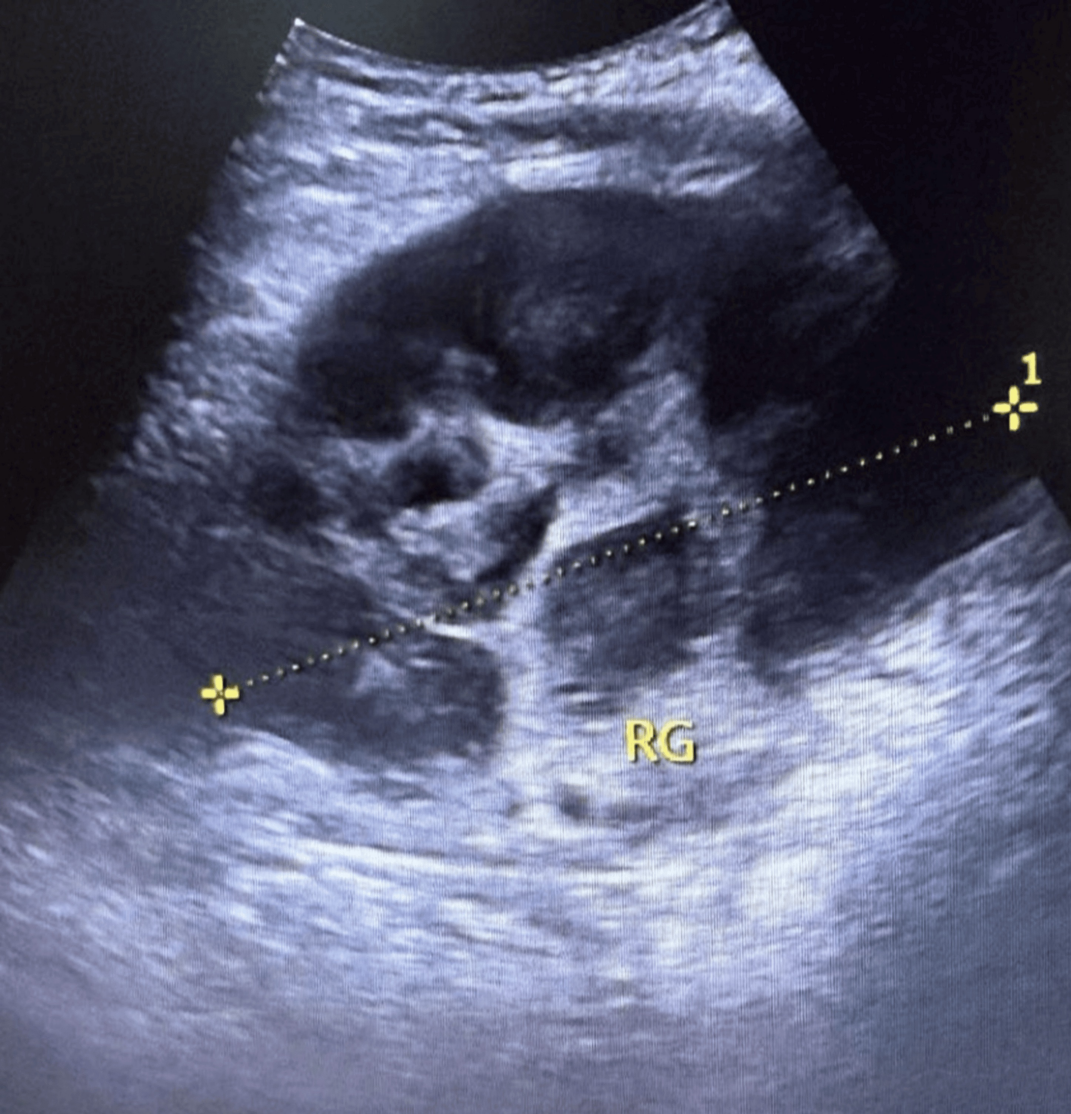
Ultrasound of the left kidney demonstrating renal enlargement with poor corticomedullary differentiation, without evidence of calculi.
